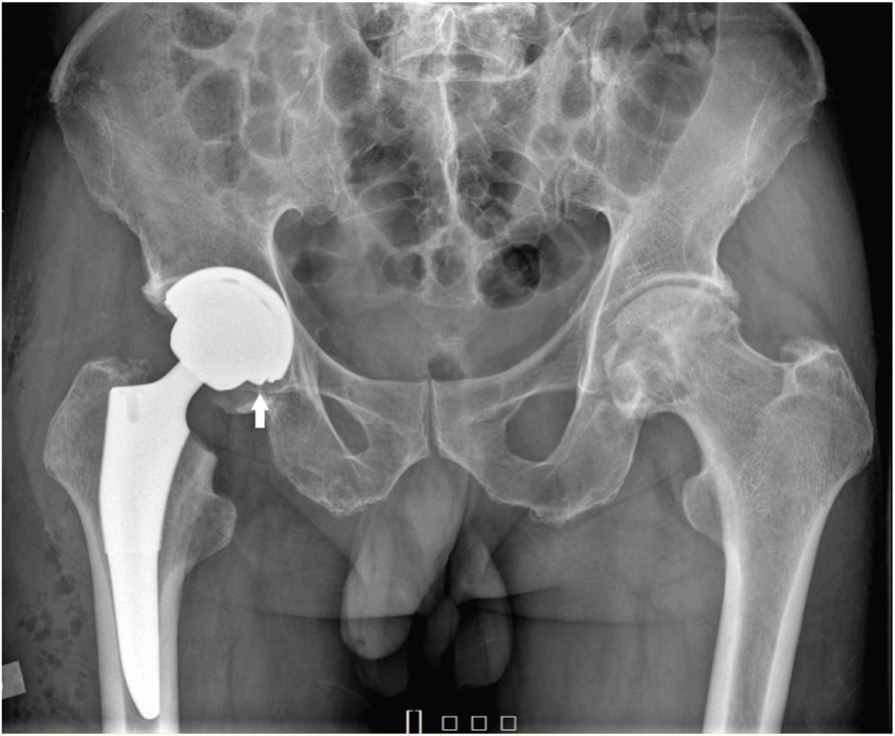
Plain radiograph and computed tomography of pelvis immediately after the surgery. A protruding ceramic liner rim can be observed on a plain hip radiograph the day after THA. (arrow)
